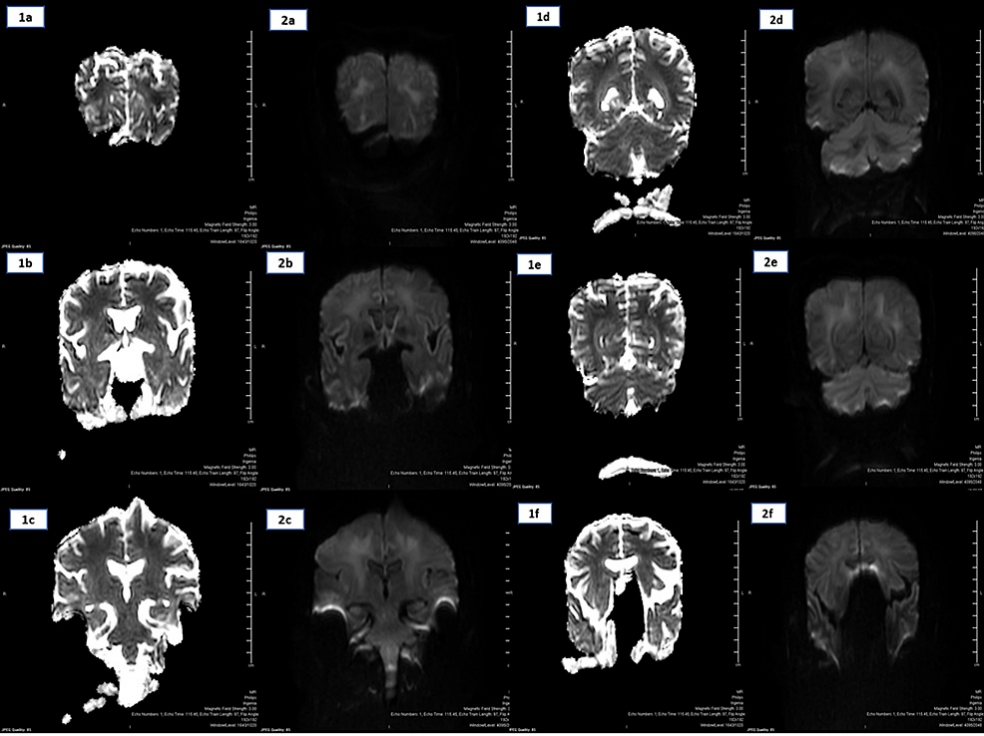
(1a-1f): Apparent diffusion coefficient (ADC) map arrow showing bilateral symmetrical supratentorial diffusion restriction in the centrum semiovale and occipital subcortical regions. (2a-2f) Coronal MRI diffusion-weighted imaging showing bilateral symmetrical supratentorial diffusion restriction in the centrum semiovale and occipital subcortical regions.
